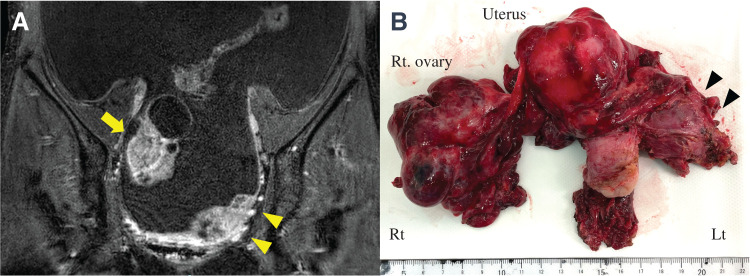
Preoperative imaging and gross surgical findings of recurrent peritoneal dissemination of ovarian angiosarcoma. (A) Pelvic MRI reveals diffuse peritoneal dissemination involving the left pouch of Douglas (arrowheads), uterine serosa, and right adnexa (arrow). (B) Macroscopic view of the resected uterus, right adnexa, and peritoneal lesions at the left pouch of Douglas (arrowheads) showing multiple hemorrhagic nodules consistent with disseminated angiosarcoma.
